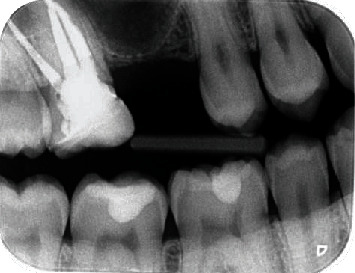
Bitewing radiography. Initial situation.
